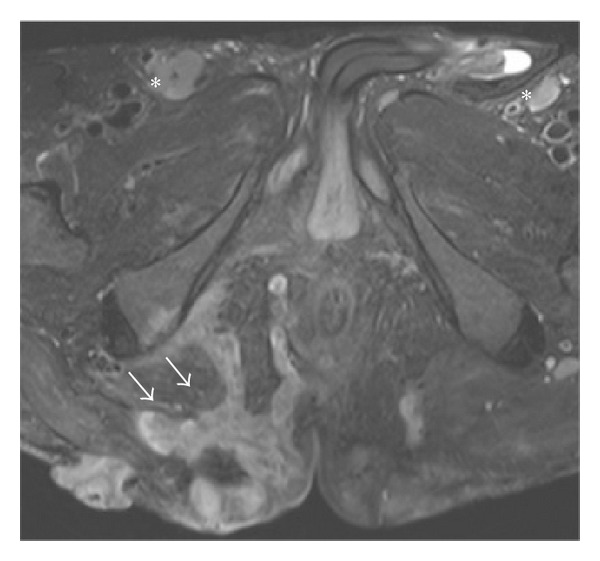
Axial FSE T2-weighted fat-suppressed image at presentation shows complex right gluteal subcutaneous collections with heterogeneous high T2-weighted signal intensity and low T1-weighted signal intensity (not shown). The tubular collections (white arrows) are reminiscent of branching primary and secondary sinus tracts and probably represent the chronic phase of these suppurative inflammatory tracts, extending to the deep right ischiorectal and ischioanal spaces. Bilateral dermal thickening and induration are seen. Bilateral enlarged inguinal lymph nodes are also demonstrated (∗).
